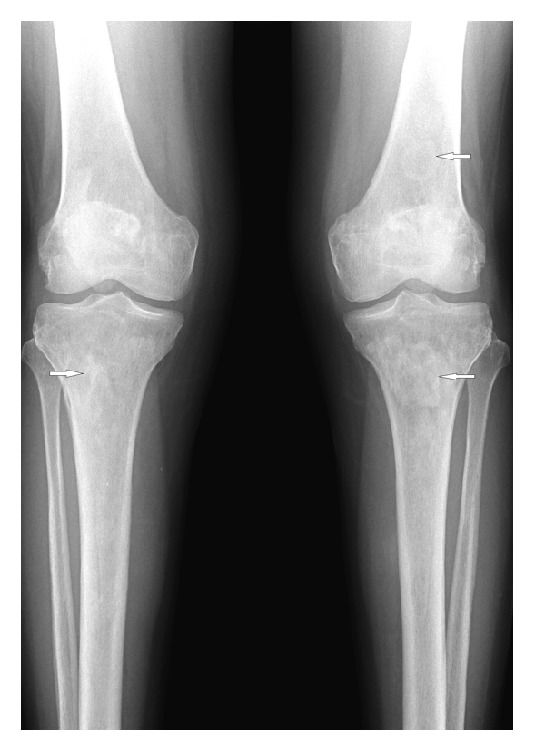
X-ray of both knees in anteroposterior view showing bilateral patchy high density donating sclerosis affecting the metaphysis of the distal femur and the proximal tibia bilaterally.
